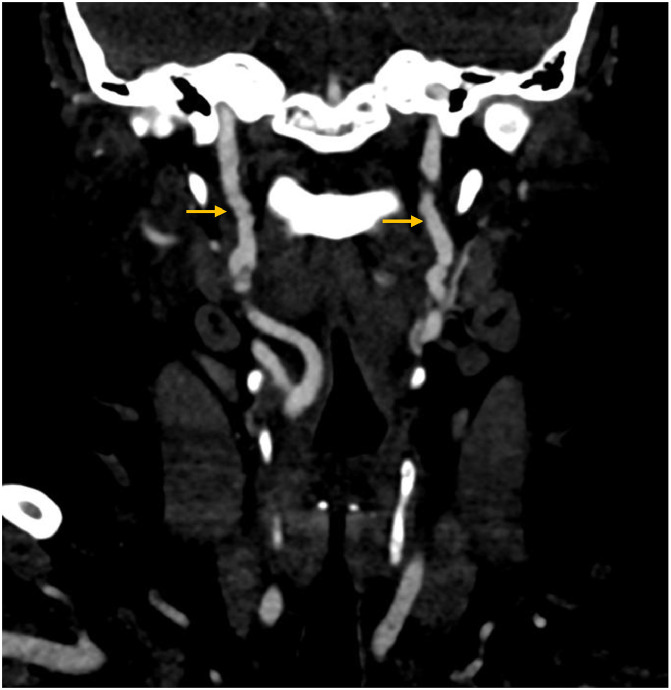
Computed tomography angiography coronal reformates, shows both internal carotid arteries are tortuous giving the string of beads appearance. No vessel occlusion.
